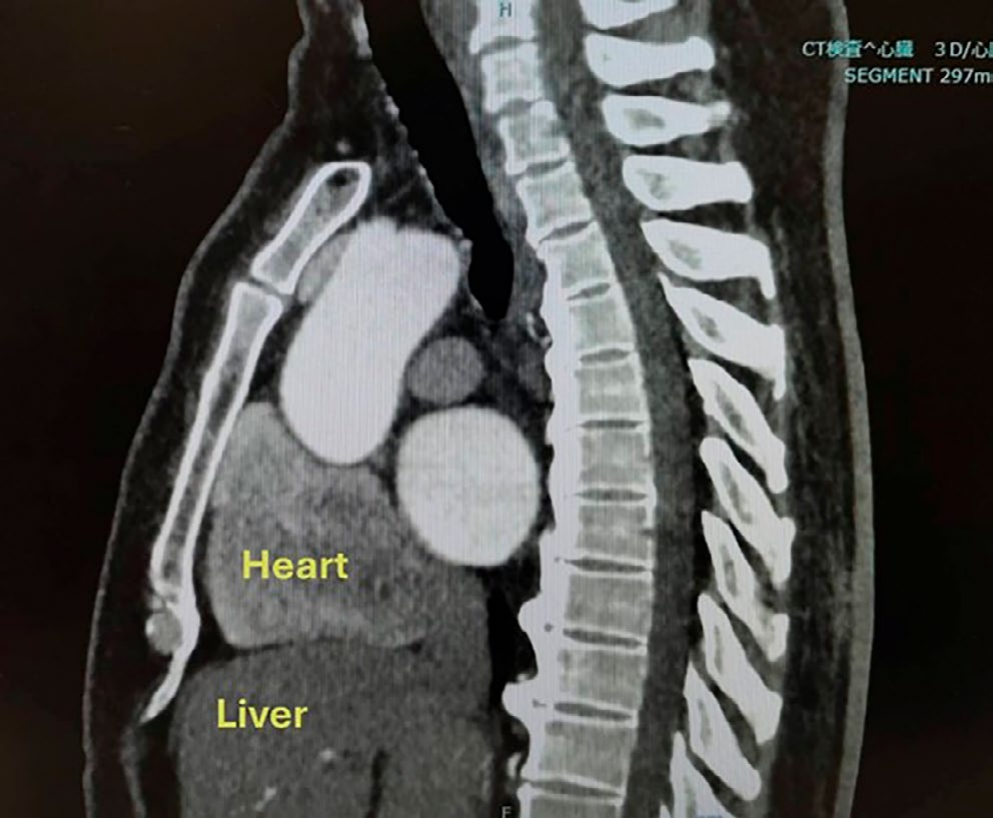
Sagittal computed tomography (CT) image at the subxiphoid level demonstrating a representative case where the liver overlaps the subxiphoid trajectory to the epicardial space.
